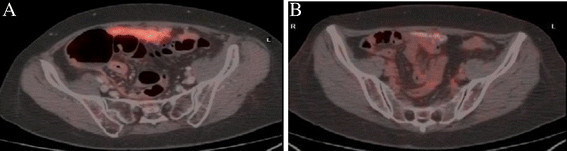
Peritoneal metastasis from ovarian cancer close to the ascending colon detected by^18^ F-FDG PET/Contrast enhanced (Ce)CT before starting bevacizumab (A) and at best response (B).
